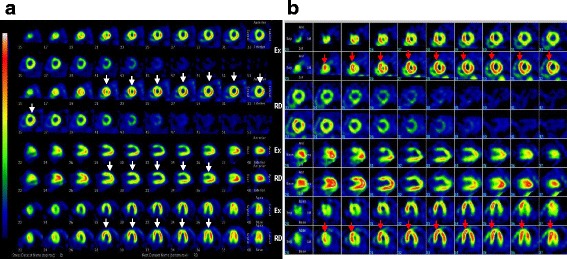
Scintigraphy before and after the redo coronary artery bypass. a Exercise stress myocardial perfusion scintigraphy using technetium tetrofosmin reveals an extensive area of ischemia on the anterior *left* ventricular wall before redo coronary artery bypass grafting (*white arrows*). b Adenosine triphosphate stress myocardial perfusion scintigraphy using technetium tetrofosmin reveals blood flow recovery in the intraventricular septum after the redo coronary artery bypass grafting (*red arrows*)
